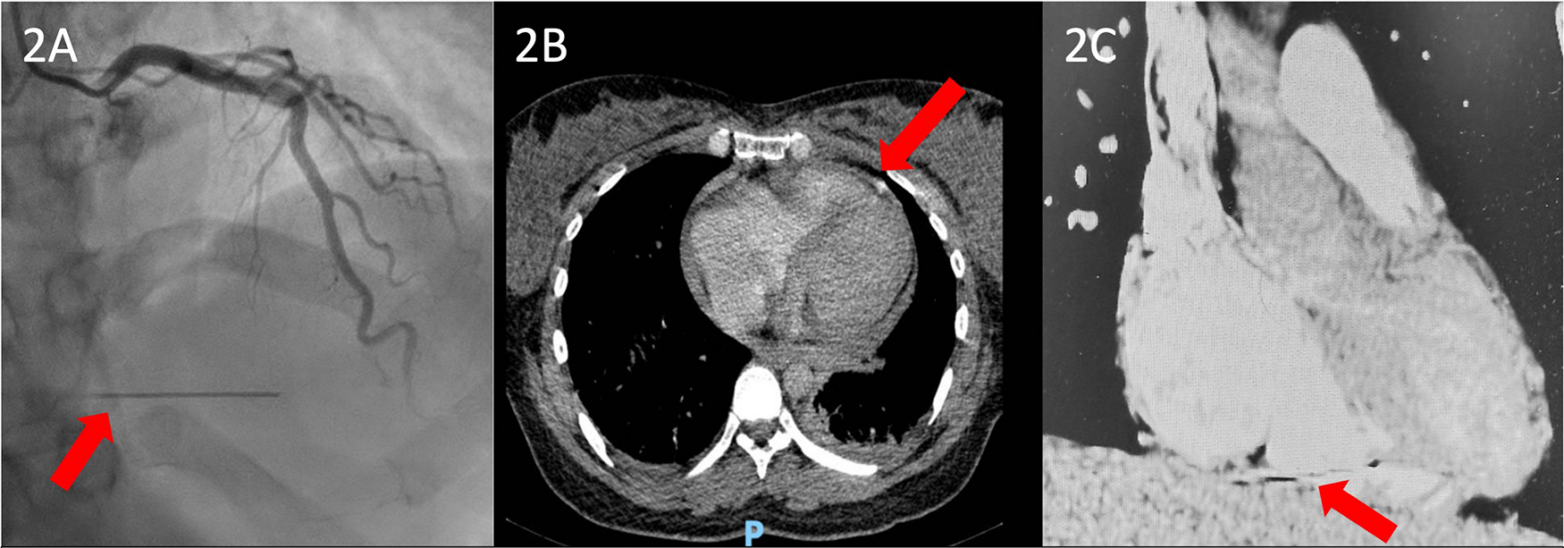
(A) Preoperative coronary angiography showing an unusual hyperdense radiopaque foreign body likely embedded within the myocardial wall, with synchronous motion with the cardiac cycle (red arrow). (B) Coronal CT scan view and (C) sagittal CT scan view showing a hyperdense needle-like foreign body containing internal lumina entrapped in the right ventricular myocardial wall.
